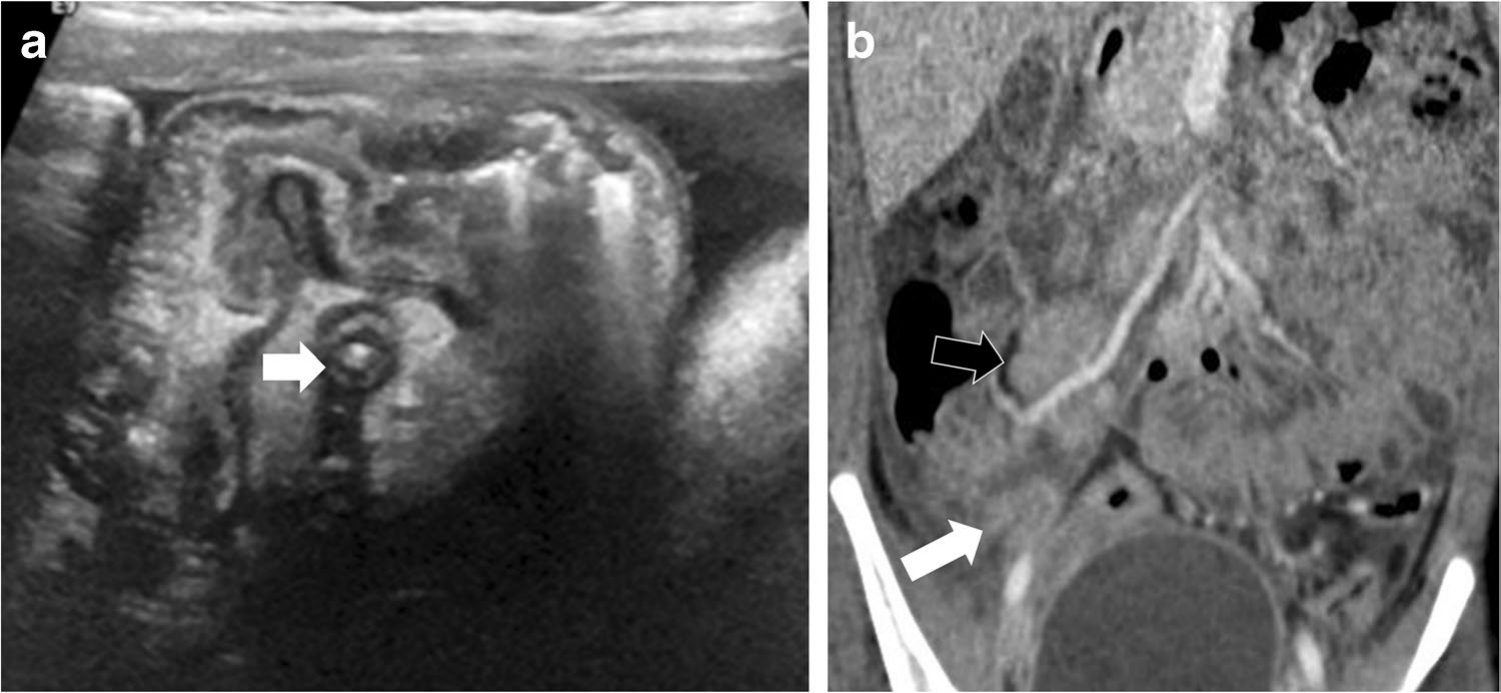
Case 4: An 8-year-old girl presented with abdominal pain, fever, and vomiting. a Initial transverse US section of right iliac fossa on presentation shows inflamed appendix (*arrow*) with inflammatory changes. Findings were suspicious for appendicitis. b CT scan (coronal post-contrast) shows multiple enlarged ileocolic lymph nodes (*black arrow*), largest 20 mm in short axis, free fluid in right iliac fossa, inflamed mesentery, ileal and cecal thickening and inflamed appendix (8 mm, *white arrow*)
